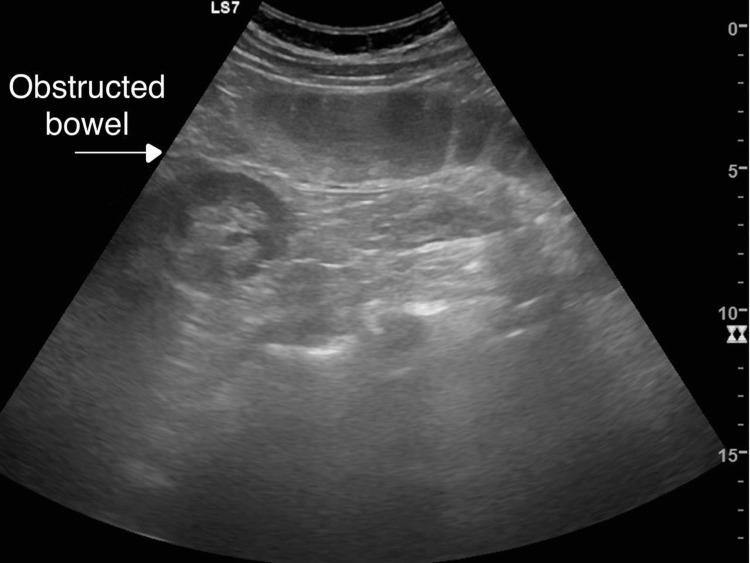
Ultrasound image demonstrating an obstructed bowel.
